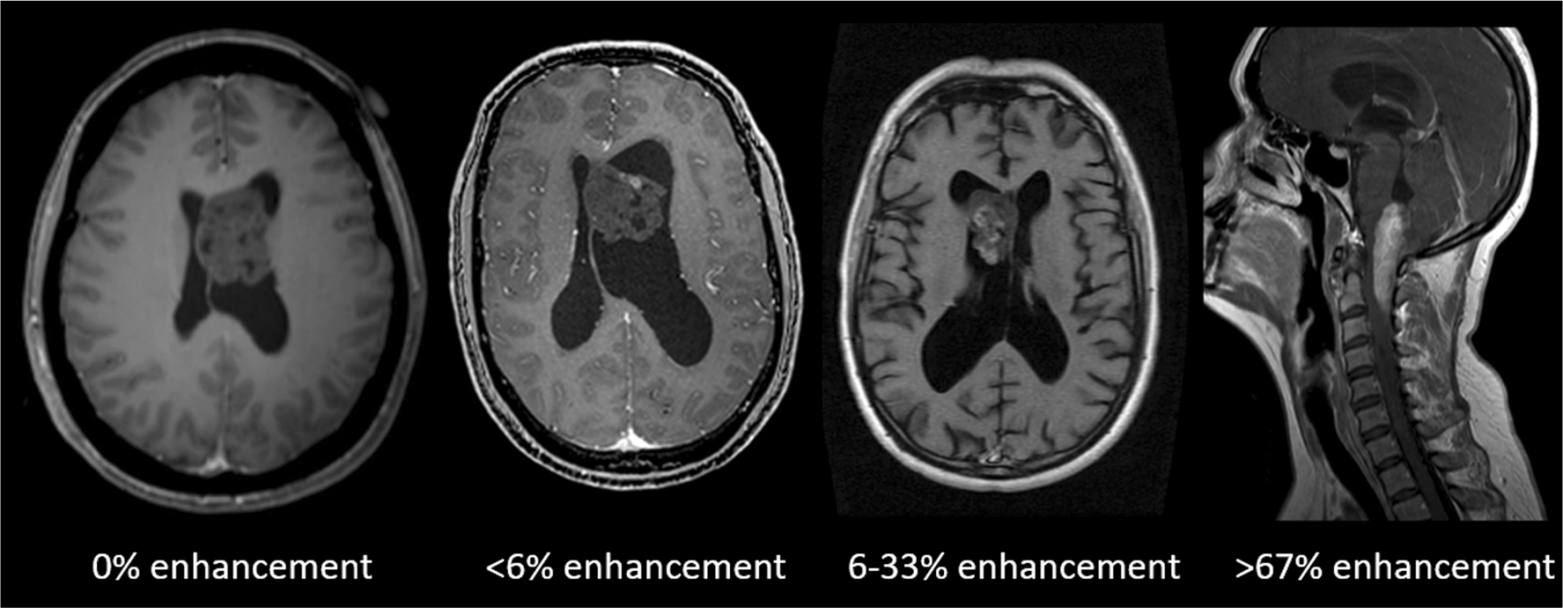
Representative case examples of varying degrees of proportional contrast enhancement on post-gadolinium contrast T1-weighted imaging
